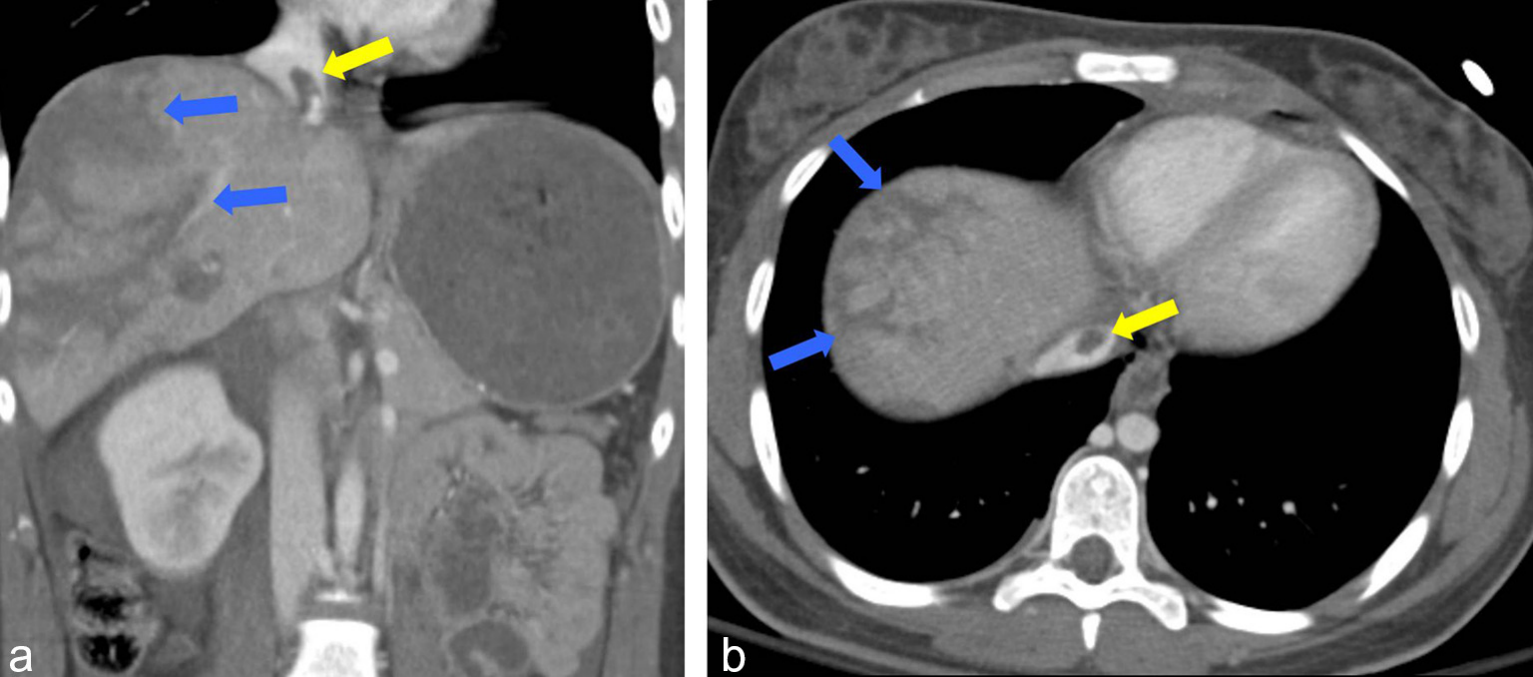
Contrast-enhanced CT abdomen coronal (a) and axial (b) reformats demonstrate the inferior vena cava thrombus (yellow arrow) and the hepatic lacerations (blue arrows).
